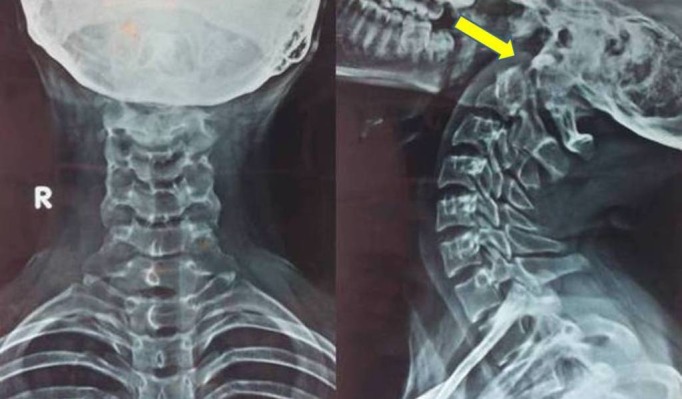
X-Ray Anteroposterior View (left) and Lateral View (right) of the Cervical Spine Subluxation of the C1 vertebra over the C2 vertebra (arrow).
